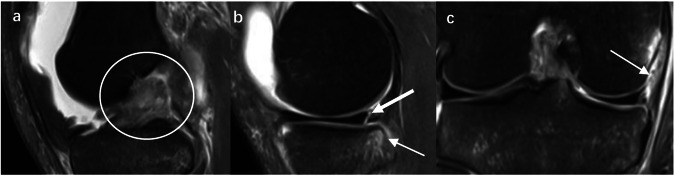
Diagnosis of aACL rupture combined with ramp lesions on MRI. A 27-year-old male sprained right knee while playing basketball with knee pain for 5 days. Sagittal and coronal IM-WI MR images with fat saturation showed acute ACL complete rupture (a, circle); the complete fluid filling signal at the meniscocapsular junction of PHMM (b, bold arrow); the bone contusion on the posterior of MTP (b, pinpointed arrow) and swelling of the meniscofemoral ligament (c, arrow). PHMM, posterior horn of the medial meniscus; MTP, medial tibial plateau; IMWI, intermediate weighted image
